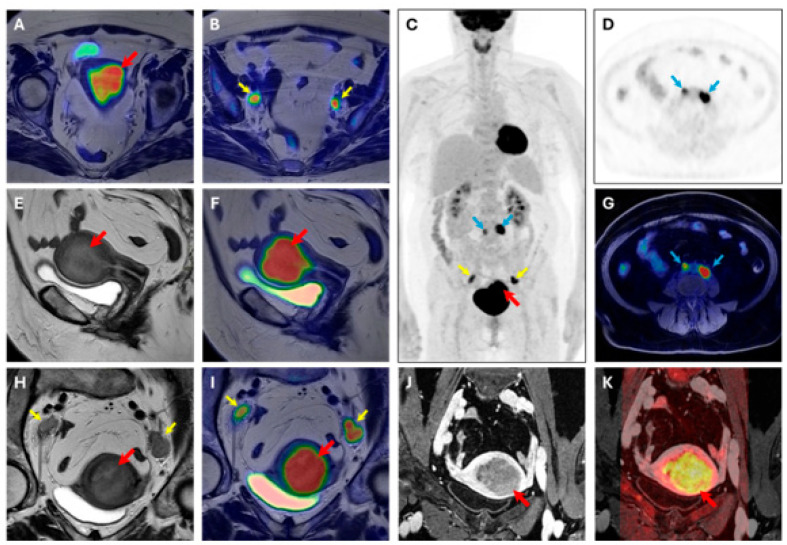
[^18^F]FDG PET/MRI scan. Image of a 63-year-old woman with endometrial cancer (stage IIIC, endometrioid grade 2, LVSI positive, p53 preserved, MMRd, myometrial invasion > 50%). (A,B) axial large field-of-view T2W-PET fusion image, (C) coronal whole body PET, (D) axial PET image, (E) sagittal T2W image, (F) sagittal T2W-PET fused image, (G) axial T1 fat suppression–PET fused image, (H) axial oblique T2W image, (I) axial oblique T2W-PET fused image, (J) axial oblique T1 post-contrast agent administration, (K) axial oblique T1 post-contrast agent administration-DWI b1000 fused image. Volume index: 114 cc; TTV: 59 cc; TVR: 68%; ADCmean: 1.19 × 10^−3^ mm^2^/s; ADCmin: 0.253 × 10^−3^ mm^2^/s. PET parameters of endometrial cancer SUVmax 19.42; SUVmean:13.27; MTV: 34.15; TLG: 453.2. Red arrow: primary EC; yellow arrow: pelvic lymph node metastases; blue arrow: abdominal lymph node metastases.
